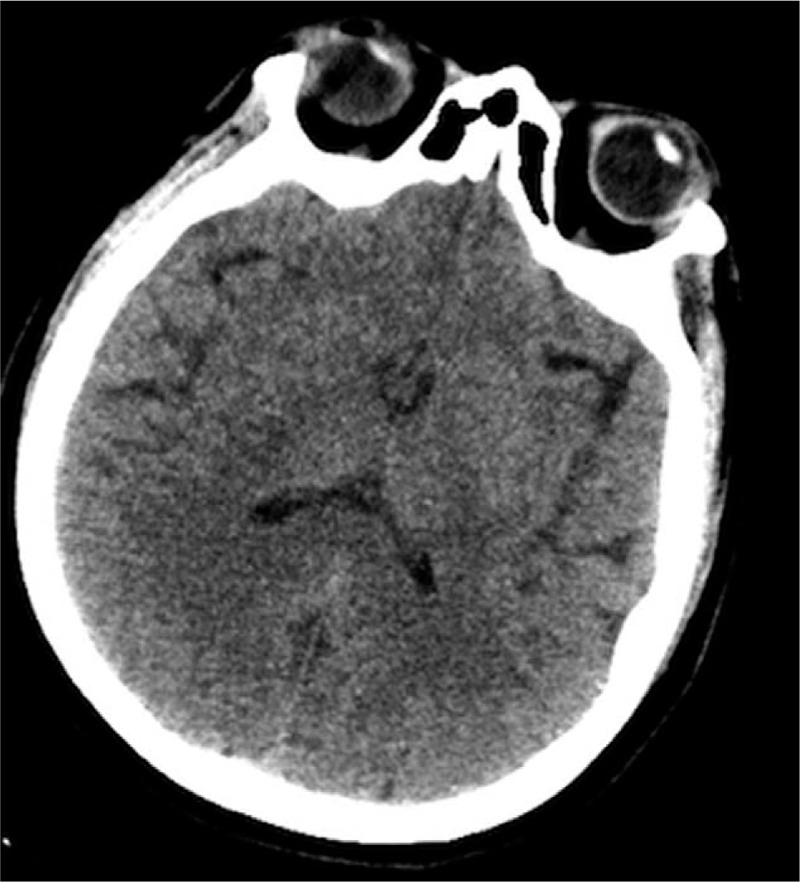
Cerebral CT scan taken 3 h after the surgical procedure showing no obvious abnormalities. CT = computed tomography.
